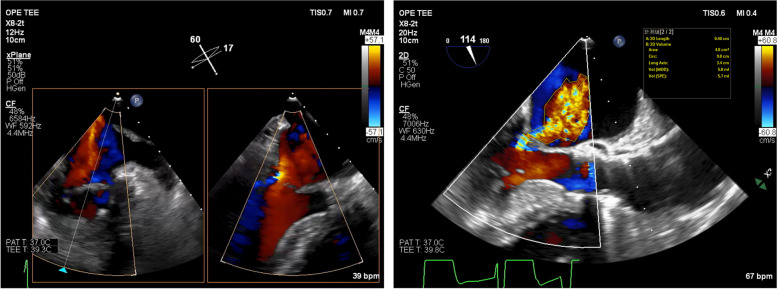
Intraoperative transesophageal echocardiography (TEE) (post-deploy). Movie. In systolic period, central regurgitation flow was detected in indentation of mitral valve with color Doppler mode. Left side view showed mid-commissural view. Regurgitant flow of different time phases are shown. LV long axis view. Vena contracta was 4.0mm. This semi-quantification revealed mild to moderate regurgitation
